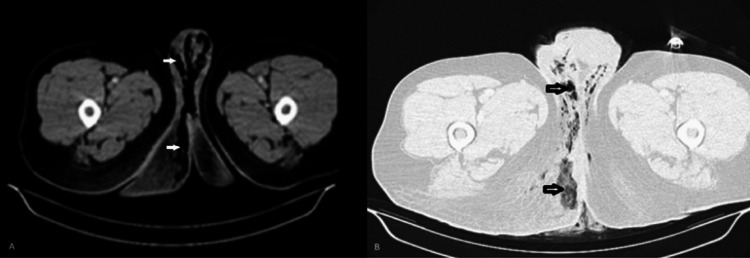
A. Axial CT scan of the perianal region. The white arrows indicate the presence of diffuse gas within the perineum and soft tissues. B. Axial CT scan of the perianal region (lung window). The arrows indicate the presence of diffuse gas within the perineum and soft tissues.
